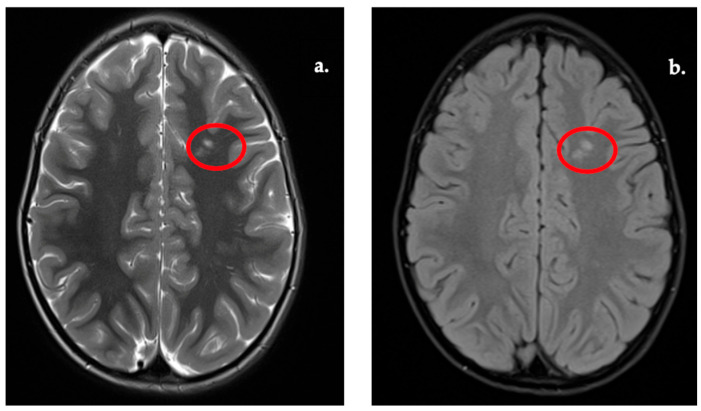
WMSA present in corona radiata. (a) T2w image. (b) FLAIR image.
